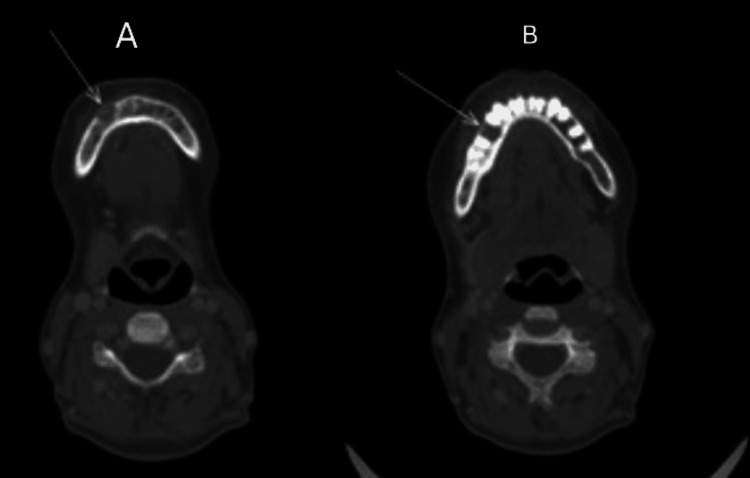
(A and B) Contrast‑enhanced CT of the right oral cavity demonstrating a 3.6 × 3.2 × 2.8 cm enhancing mass (arrows) with internal calcifications and soft‑tissue extension through the interval between the maxilla and mandibular ramus into the buccal soft tissues. CT: computed tomography
